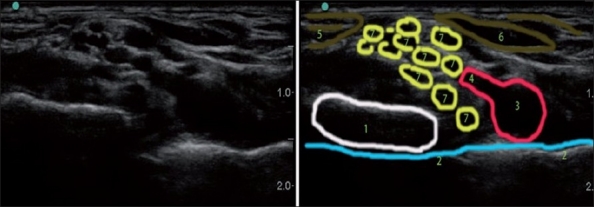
Supraclavicular ultrasound scan demonstrating the dorsal scapular artery. (1) First rib, (2) lung, (3) subclavian artery, (4) dorsal scapular artery, (5) middle scalene muscle, (6) anterior scalene muscle, (7) brachial plexus
